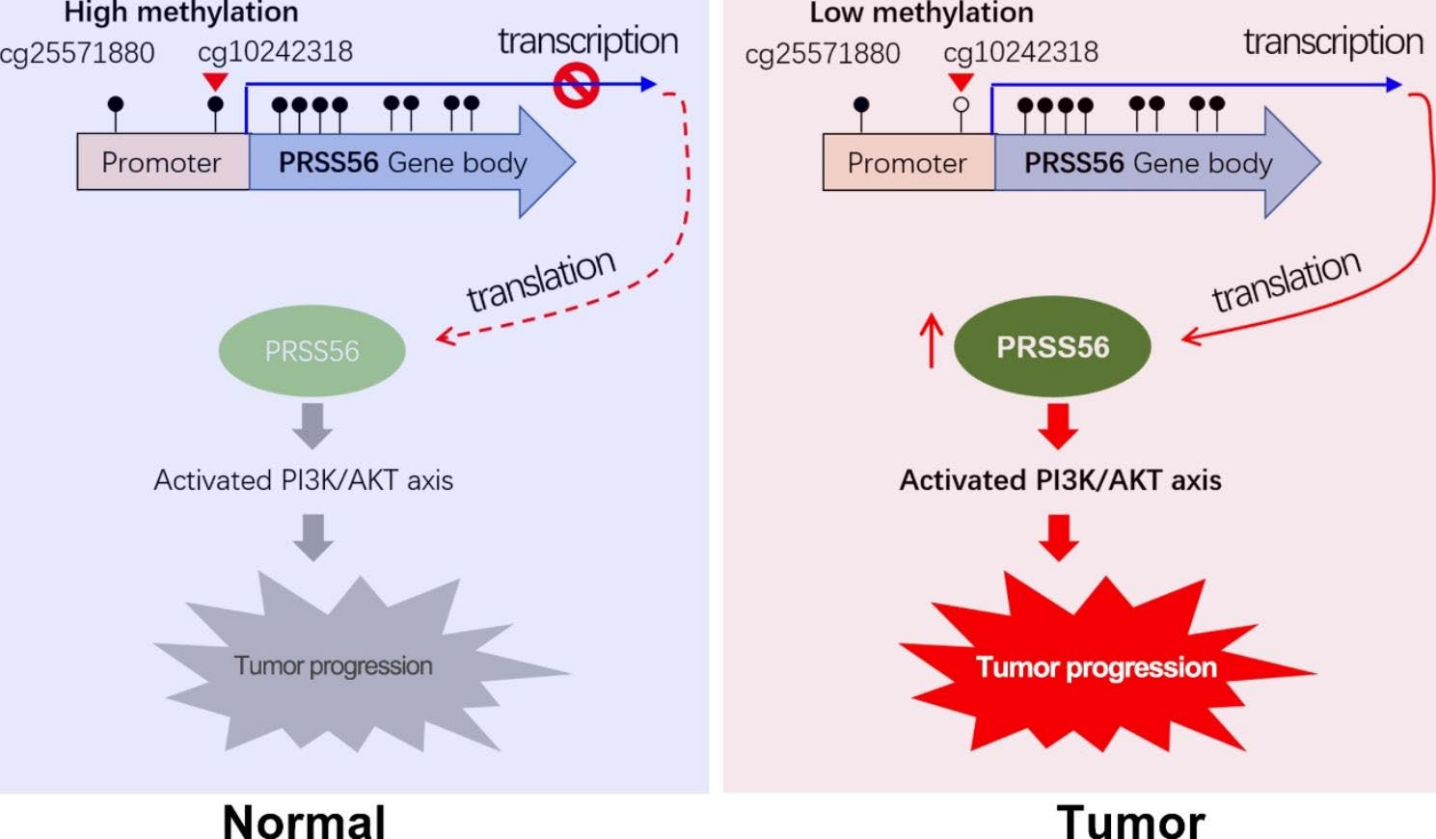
The working model of the CT antigen PRSS56 in GC and CRC. Briefly, PRSS56 was a novel CT antigen specifically expressed in normal testis, and its expression was frequently reactivated in diverse tumors, especially gastrointestinal cancer. In GC and CRC, PRSS56 overexpression was due to its reduced methylation level of PRSS56 promoter at CpG site cg10242318. In turn, PRSS56 overexpression promoted GC and CRC progression by activating PI3K/AKT axis
